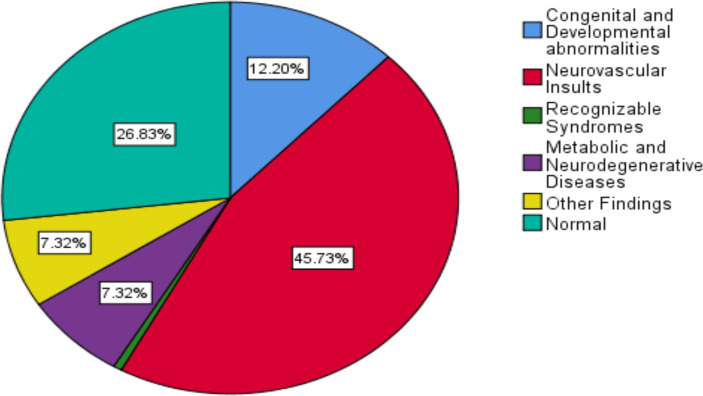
Frequency of final imaging diagnosis in 164 patients with developmental delay who came for brain MRI evaluation at TASH and at one private diagnostic center in Addis Ababa in 2019–21
